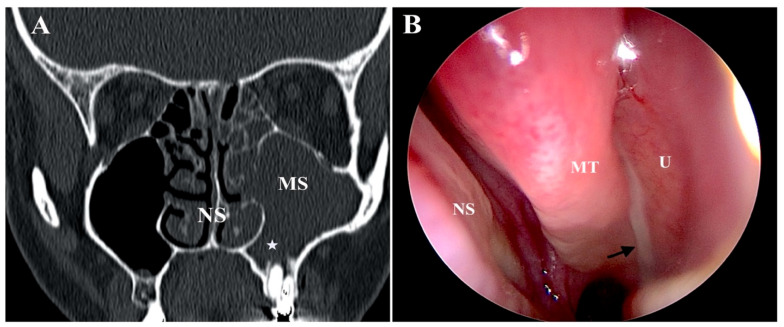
Periapical lesion with alveolar bone loss (marked with an asterisk) and total opacification of maxillary sinus (MS) with an extension to the anterior ethmoids visible on coronal CT scan (A) and pus in the middle meatus visible during nasal endoscopy (B); pus marked with a black arrow. NS—nasal septum, MT—middle turbinate, U—uncinate process.
